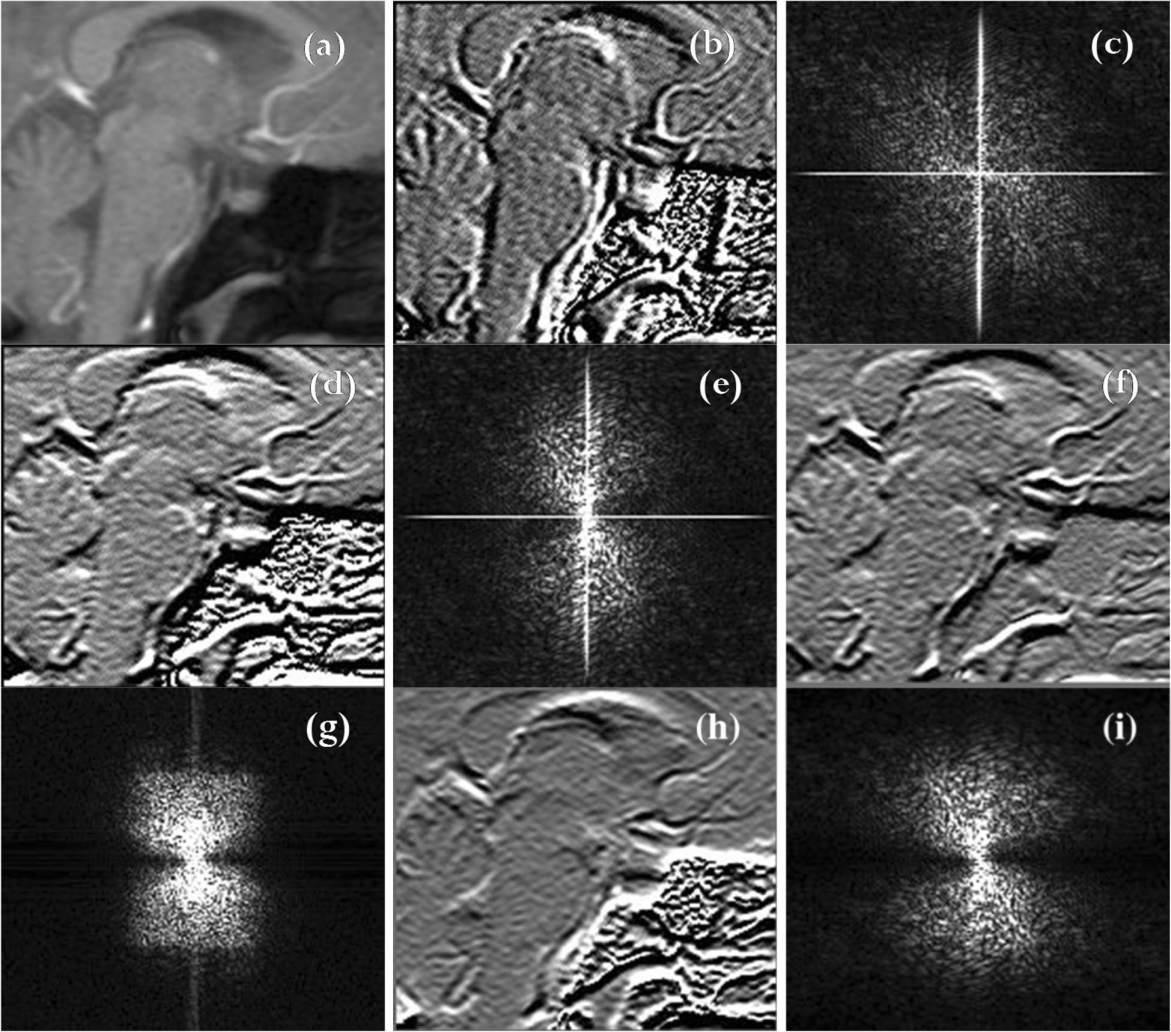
Comparison across ICF-based HPFs and traditional HPFs. (a) MRI. (b) MRI filtered with bivariate cubic polynomial model function B32D ICF-based HPF. (c) The k-space magnitude of (b). (d) MRI filtered with the bivariate cubic Lagrange polynomial model function G42D ICF-based HPF. (e) The k-space magnitude of (d). (f) MRI filtered with traditional HPF. (g) The k-space of (f). (h) MRI filtered with the bivariate cubic B-spline H42D ICF-based HPF. (i) The k-space magnitude of (h)
